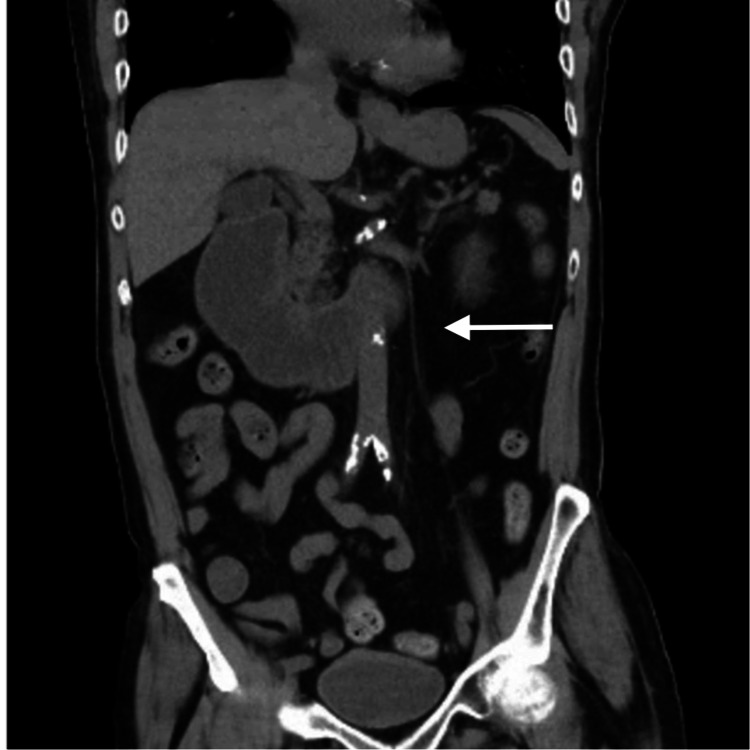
Coronal CT scan of abdomen and pelvis showing dilation of proximal small bowel up to 4.2 cm with a transition point at the superior mesenteric artery take-off CT, computed tomography
